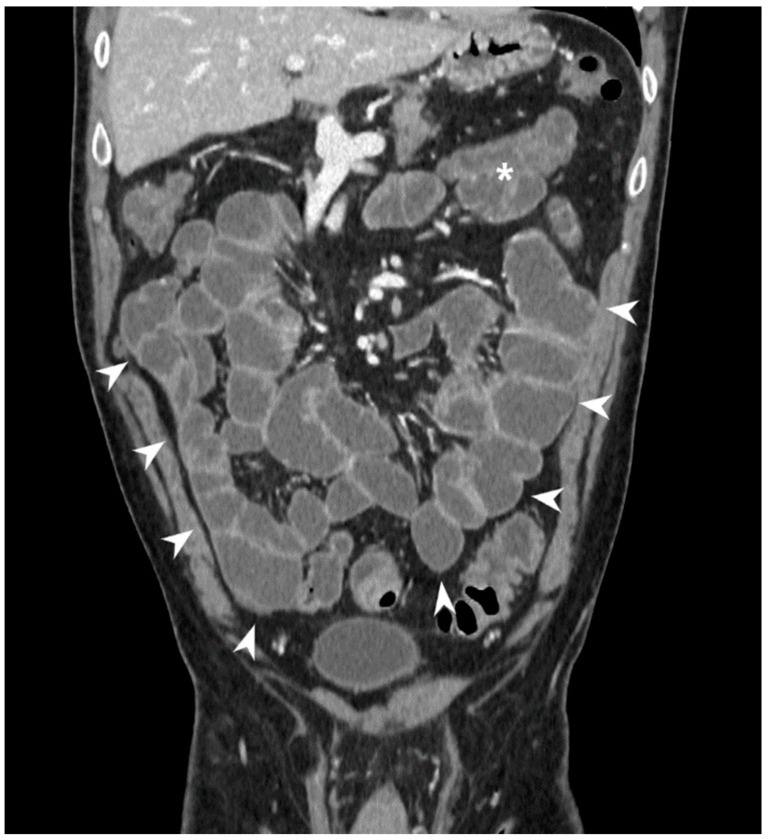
Coronal CT-E image after iodinated contrast medium injection: example of optimal distention of the ileal loops obtained by oral administration contrast medium (arrowheads); jejunal loops are on the top left (white asterisk). Original figures by LMM and LL.
